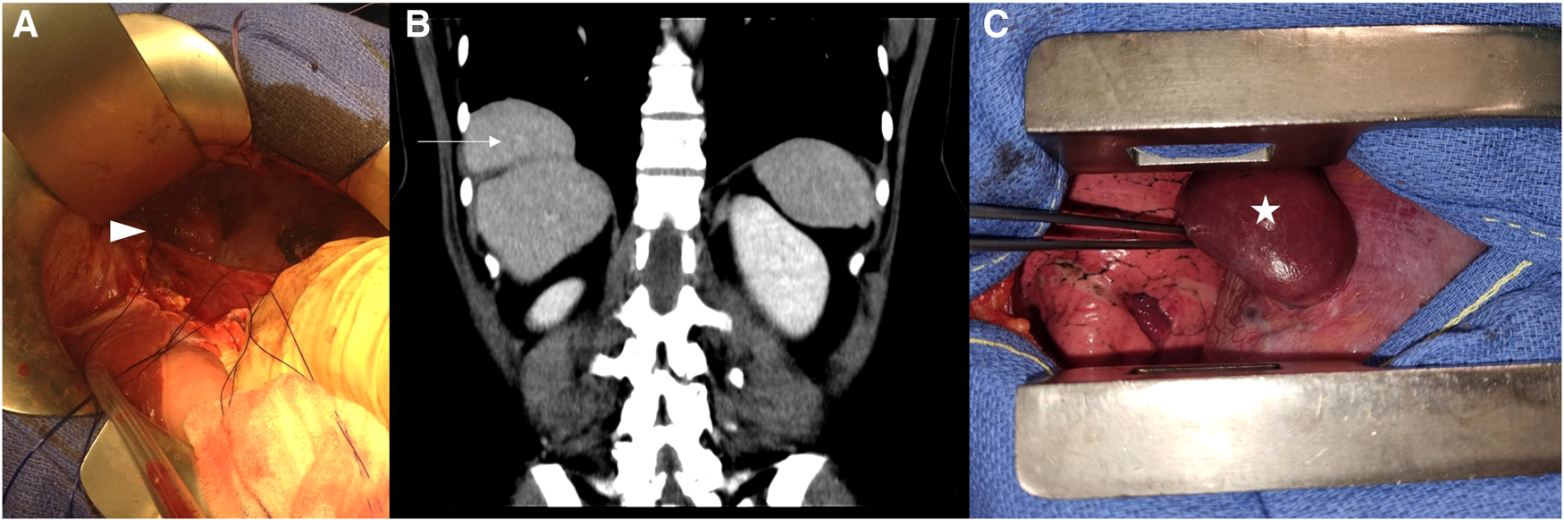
Case 3: (A) Upper midline laparotomy after the reduction of the Bochdalek hernia content. Case 4: (B) The pre-operative thoraco-abdominal CT in the frontal view is showing the “mushroom” shape of the protruding liver and (C) the per-operative view following right thoracotomy, showing the herniated liver before its reduction, presented as a “mushroom” shape.
